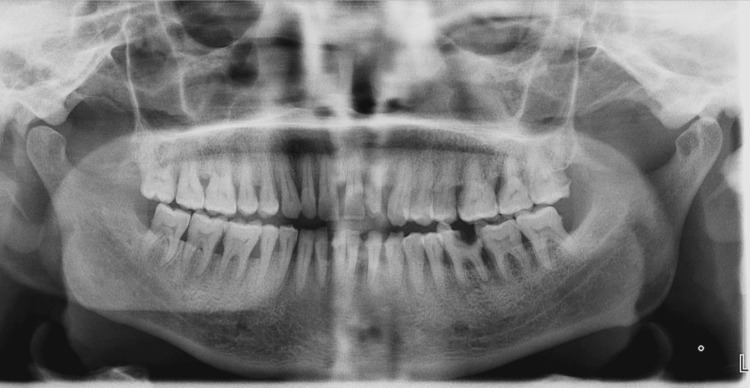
A panoramic radiograph of the patient showing multiple areas of vertical and horizontal bone loss
